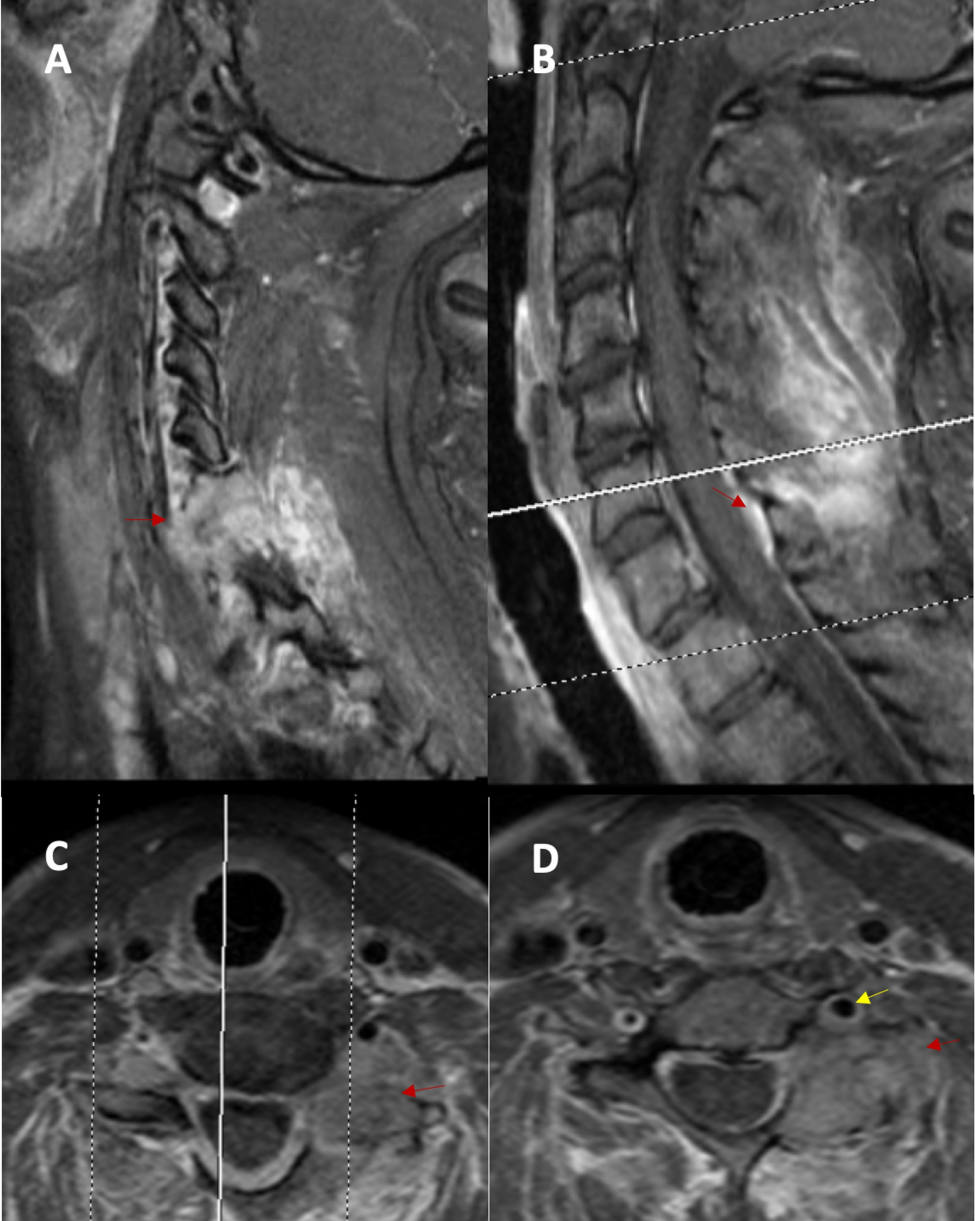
MRI cervical with and without contrast further characterizing mass (A) Left parasagittal cervical MRI with contrast demonstrating heterogeneously enhancing mass (red arrows) originating from the facets with extension into the soft tissues of the neck. (B) Sagittal MRI with contrast demonstrating extradural mass of the posterior elements with extension into the soft tissues. (C) Axial MRI with contrast at the level of C6 shows mass involvement with the left facet and abuttal of the left vertebral artery. (D) Axial MRI with contrast at the level of C7 showing mass involvement of the left facet and abuttal with possible encasement of the left vertebral artery (yellow arrow) MRI: magnetic resonance imaging
